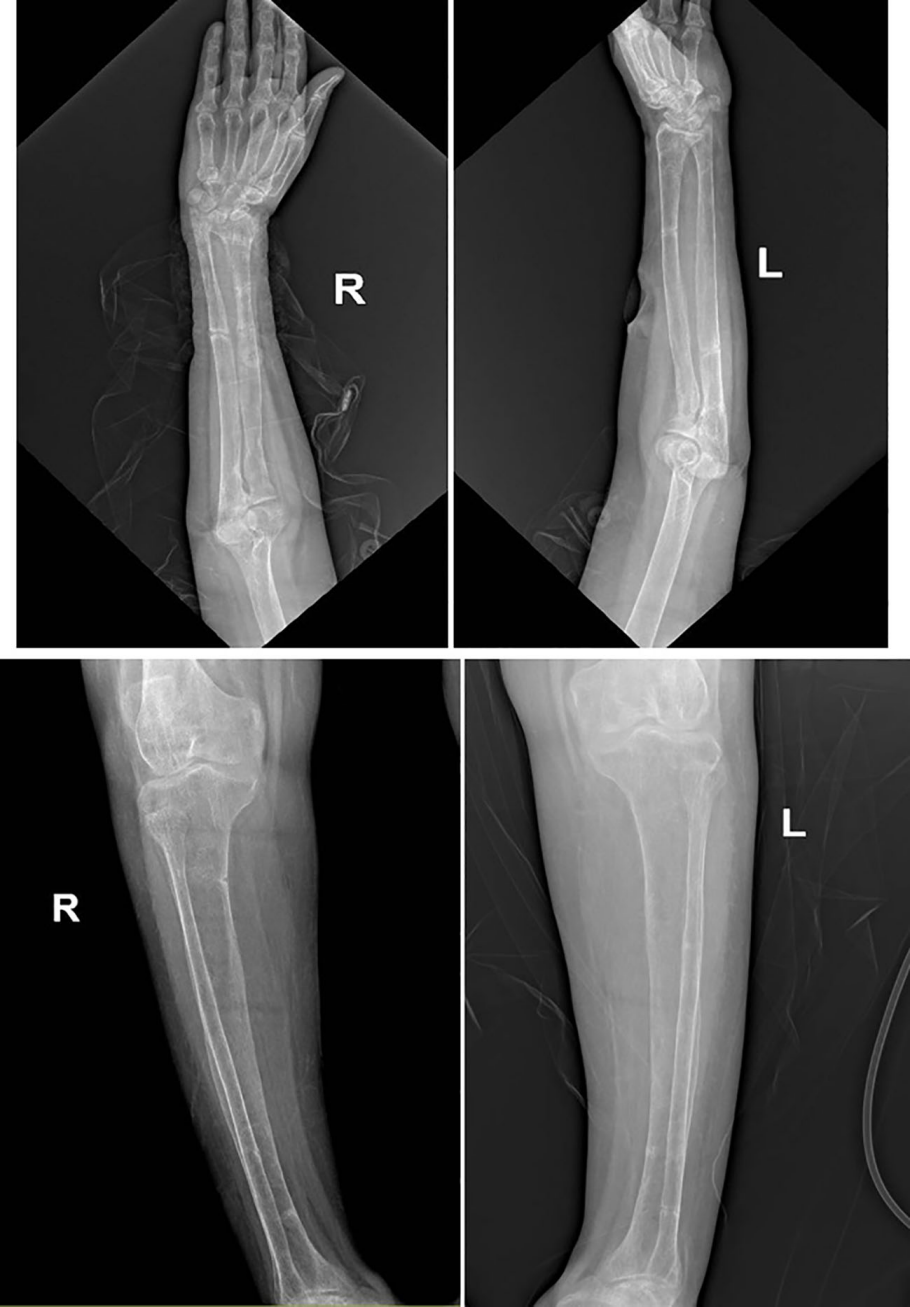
Skeletal radiographic images showing multiple fractures involving upper and lower limbs.
